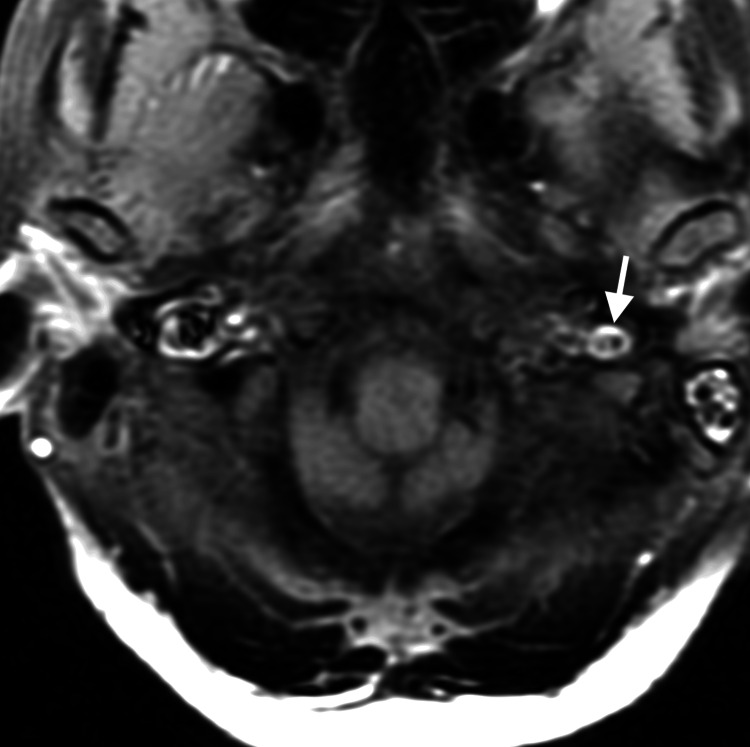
Axial T1-weighted fat-saturated non-contrast magnetization-prepared rapid gradient echo (MPRAGE) image. This shows a hyperintense crescent sign surrounding the V3 segment of the left vertebral artery with central true and false lumens (white arrow), indicating an acute intramural vertebral artery dissection based on differential arterial flow dynamics.
